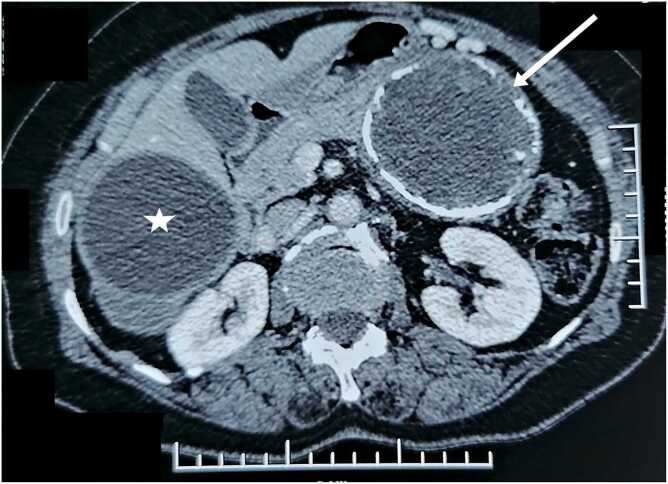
Abdominal CT scan showing the hydatid cysts of the pancreas (arrow) and the liver (star).
